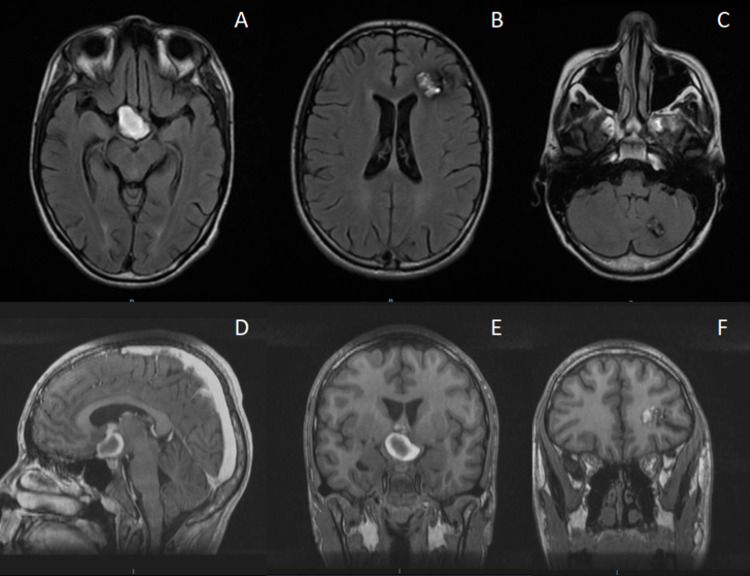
Imaging studies two years before coming to us. A-C, axial T2-FLAIR MRI shows multiple cavernous malformations, hypothalamic, cerebellar, and frontal lesions (left to right). D-E, hypothalamic cavernous malformation in different T1-weighted MRI projections, sagittal and coronal (left to right). F, frontal cavernous malformation on a coronal T1-weighted MRI
